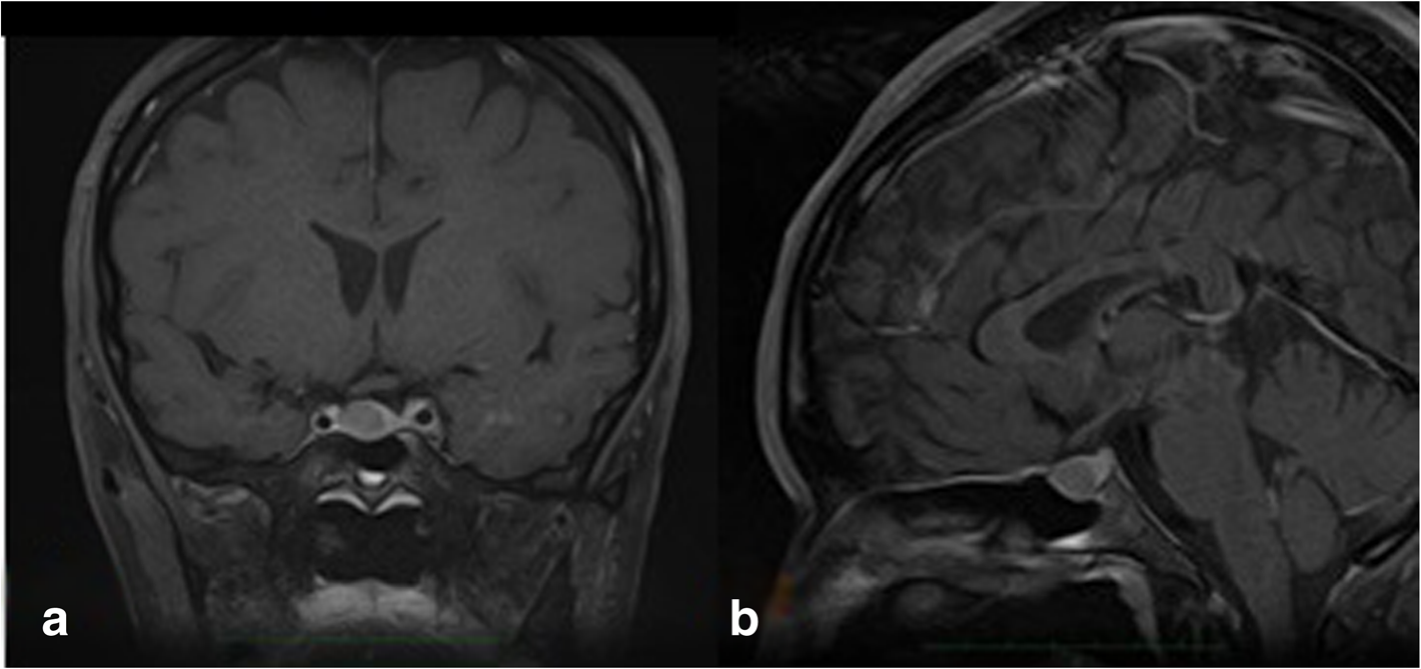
Imaging study. a T1-gadolinium magnetic resonance imaging on admission showing a less enhanced area in the sella turcica, which seemed to be a macroadenoma (10×13 mm). b The tumor slightly invaded the right lateral cavernous sinus
